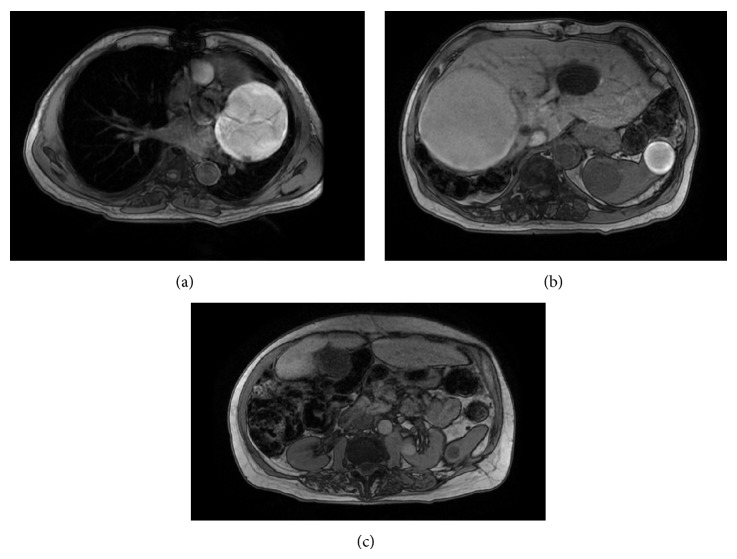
MRI of the abdomen with/without contrast (04-01-2011). (a) A heterogeneous mass is again seen adjacent to the left heart border, measuring approximately 8.5 × 8.0 cm which is partially visualized. Again, a vascular lesion is not excluded. (b) Multiple hepatic cysts are again demonstrated. A large mixed signal mass is again identified in the right lobe of the liver, which in the current study measures 13.0 × 11.7 cm. This measured 19 × 15 cm in the prior study and appears smaller in the current study. Multiple cystic lesions are again noted in the spleen. There is a mass within the spleen anteriorly which again demonstrates a peripheral rim of hypointensity, measuring approximately 3.5 × 3.6 cm. Multiple splenic cysts are noted. (c) Multiple renal cysts are present. There is a new well-circumscribed lesion in the mid pole of the left kidney measuring 1.7 × 1.6 cm which is T1 hyperintense and vaguely T2 hypointense. The subtraction image demonstrated no significant enhancement in this region. This likely represents a hyperdense cyst. There are several T1 hyperintense lesions within the kidneys bilaterally, which are too small to characterize. There are several nonenhancing T2 hyperintensities identified that appear to be within the pancreas; however, these are difficult to evaluate due to overlapping soft tissue structures in this region.
